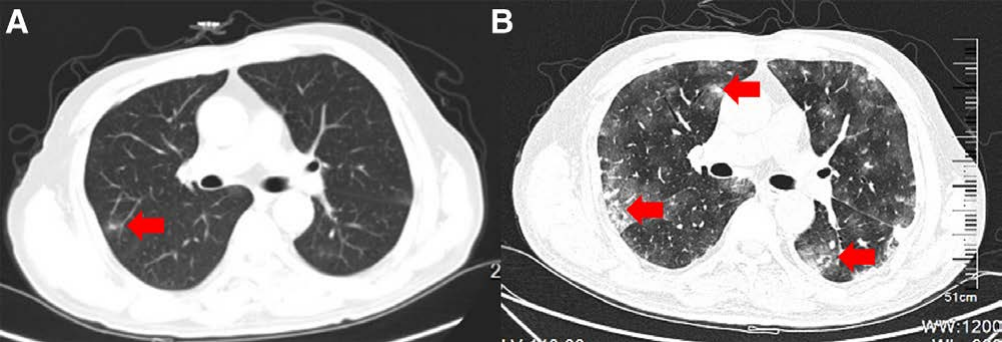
On October 26, a chest CT showed newly formed ground-glass opacities (A). On November 14, multifocal nodular high-density shadows and patchy ground-glass opacities were observed in both lungs (B). As indicated by the red marks, at the same level, both the extent of the lesion and the severity of pulmonary edema have significantly worsened between the 2 CT examinations. CT = computerized tomography.
